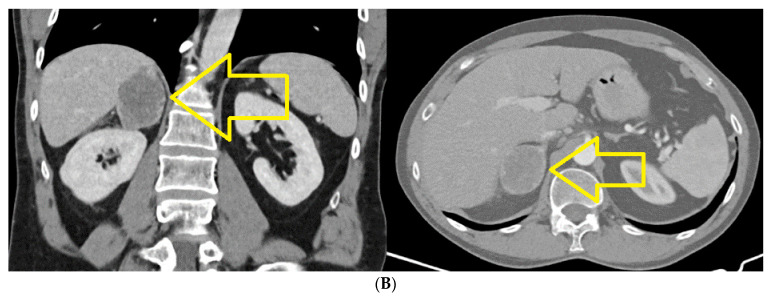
(A) Chest CT January 2022: Left upper lobe pulmonary tumor mass in dimensional progression. Small mediastino-hilar lymph nodes, not significantly changed, compared to the previous examination. (B) CT abdomen January 2022: Right adrenal secondary determination, in clear dimensional progression, newly appeared left adrenal secondary determination.
